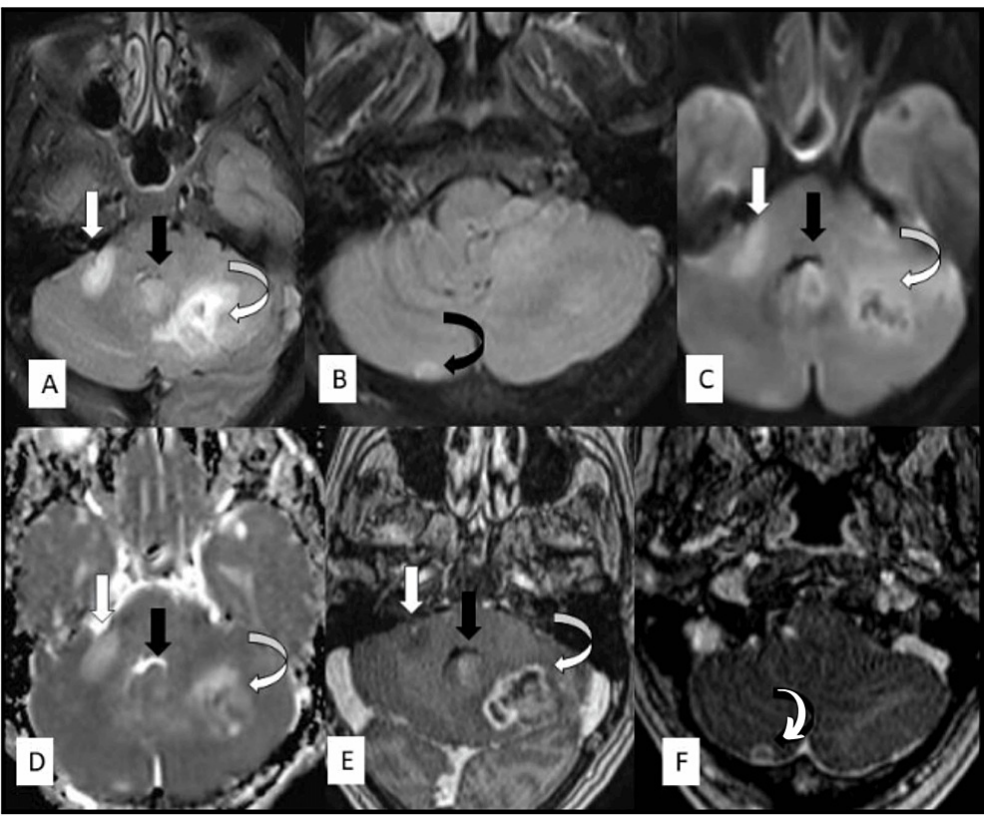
MRI axial T2 image (A) and T2 FLAIR image (B) show three T2 hyperintense lesions in right middle cerebellar peduncle (white arrow), vermis (white arrow), left cerebellar hemisphere (curved white arrow), and right cerebellar hemisphere (curved black arrow). Axial DWI (C) and ADC map (D) show restricted diffusion in the left cerebellar and vermis lesions. Axial T1 post-contrast images (E,F) show heterogenous enhancement with necrotic center in the left cerebellar lesion, peripheral enhancement in right cerebellar lesion, solid enhancement in the cerebellar vermis, and absent enhancement in the right middle cerebellar peduncle lesion. FLAIR: fluid-attenuated inversion recovery; DWI: diffusion-weighted imaging; ADC: apparent diffusion coefficient
